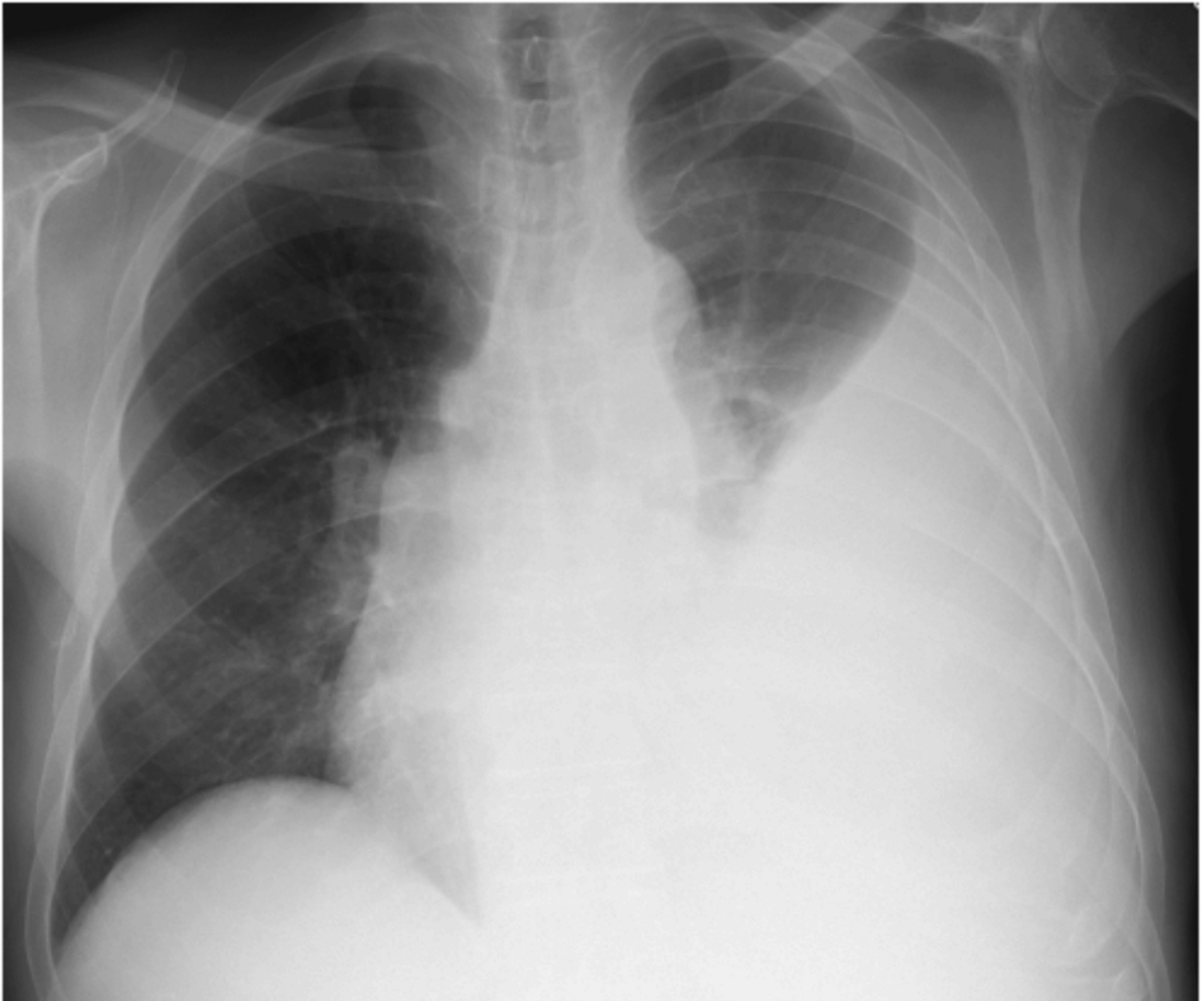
Chest radiograph (orthostatic position) showing a reduction in pleural effusion in the left lung field after thoracentesis.
